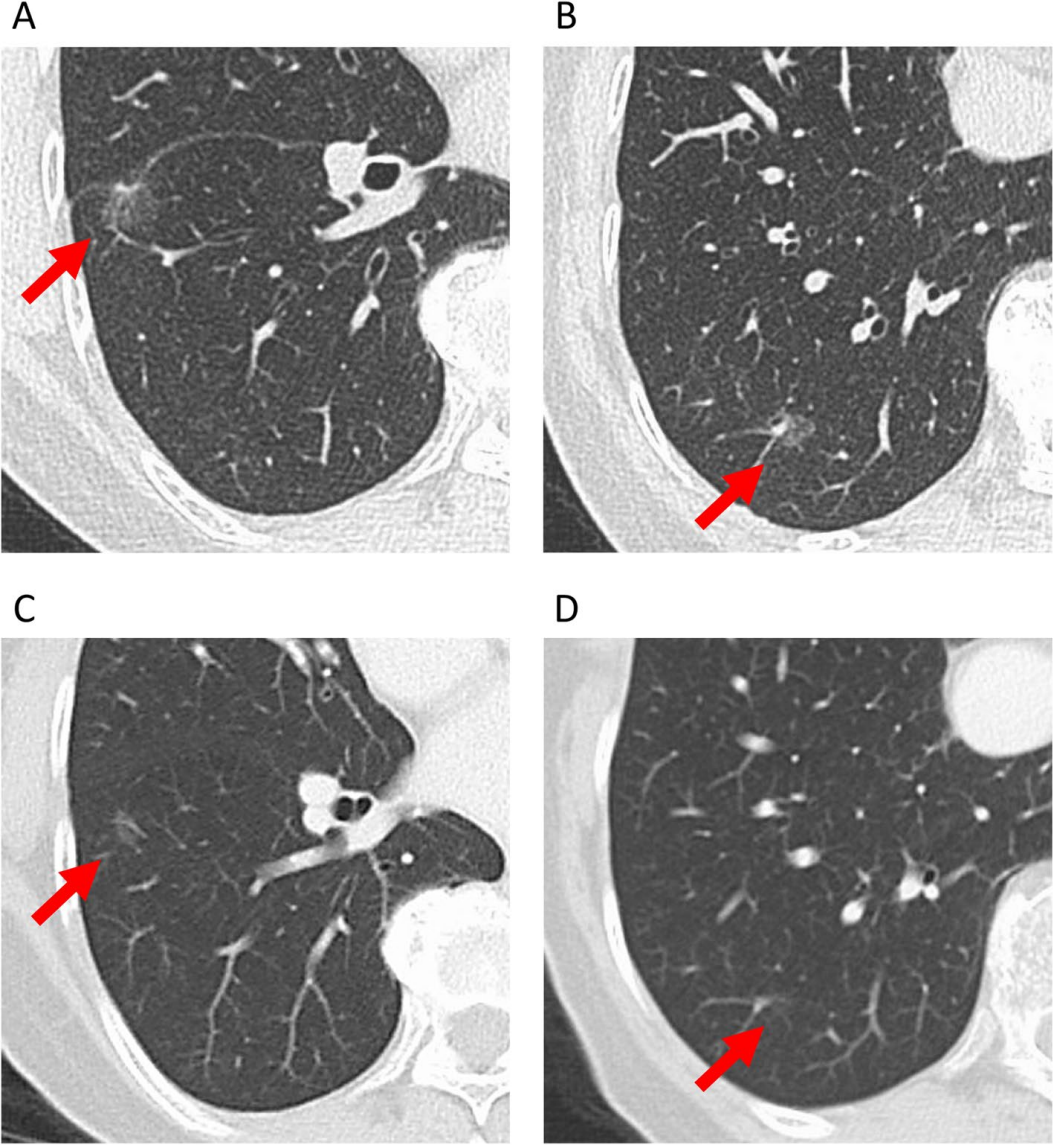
Chest CT findings. A Preoperative CT finding of the nodule in S6 segment. B Preoperative CT finding of the nodule in S10 segment. C Finding of the nodule in S6 segment on chest CT 15 months before referral. D Finding of the nodule in S6 segment on chest CT 15 months before referral
